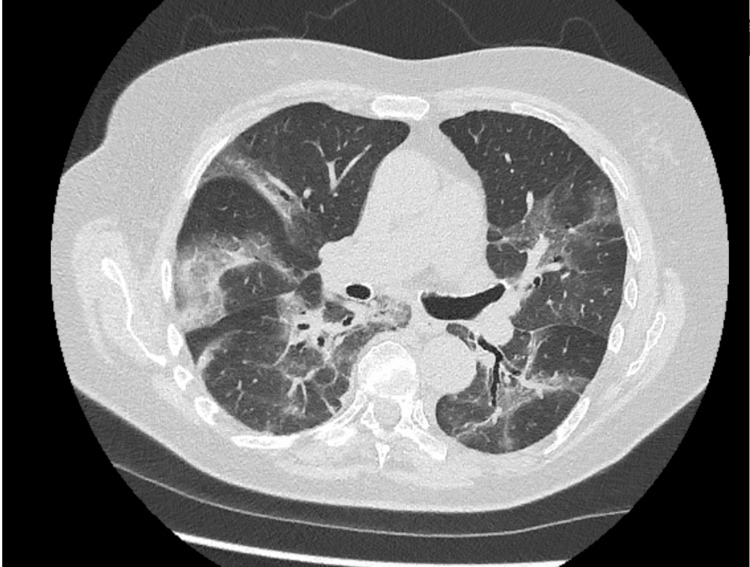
CT of the thorax on day 51 showing evolving ground-glass opacifications.
